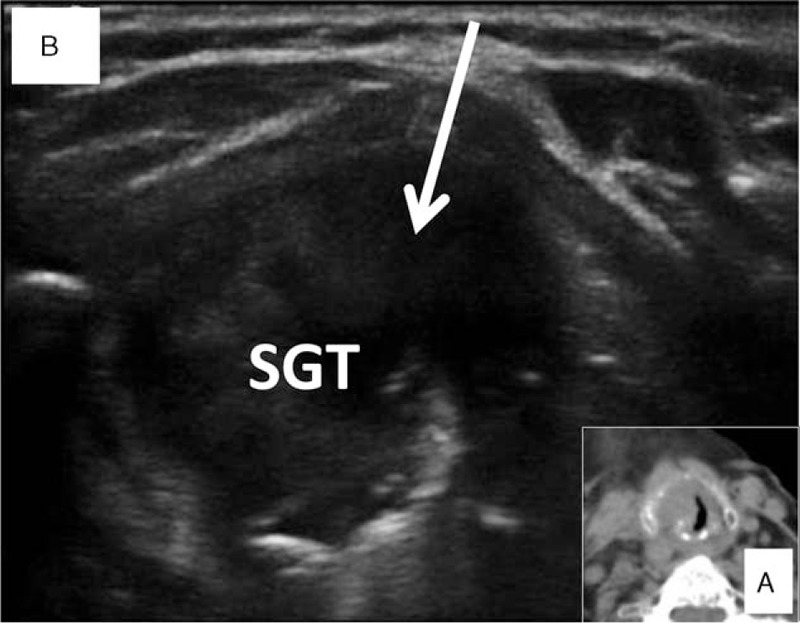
Panel A: Transverse view of the computed tomography scan shows a subglottic mass. Panel B: Ultrasound view at the level of the cricothyroid membrane shows a mass. (SGT = subglottic tumor, arrow indicates the direction of a cricothyrotomy).
